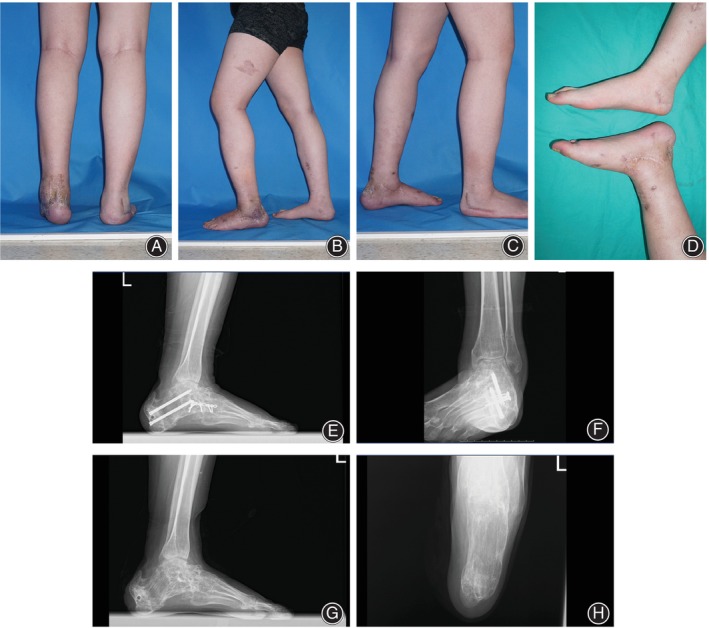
Postoperative appearance and radiography of the typical case. (A–D) The appearance of the affected foot and ankle was improved with malleolar varus and hindfoot deficiency corrected. (E–H) Fluoroscopy showed that calcaneal interface angle (CIA), angle of a longitudinal arch (ALA), metatarsal declination angle (MDA), and tibio‐calcaneal angle (TCA), as well as the length of the calcaneus, were restored.
